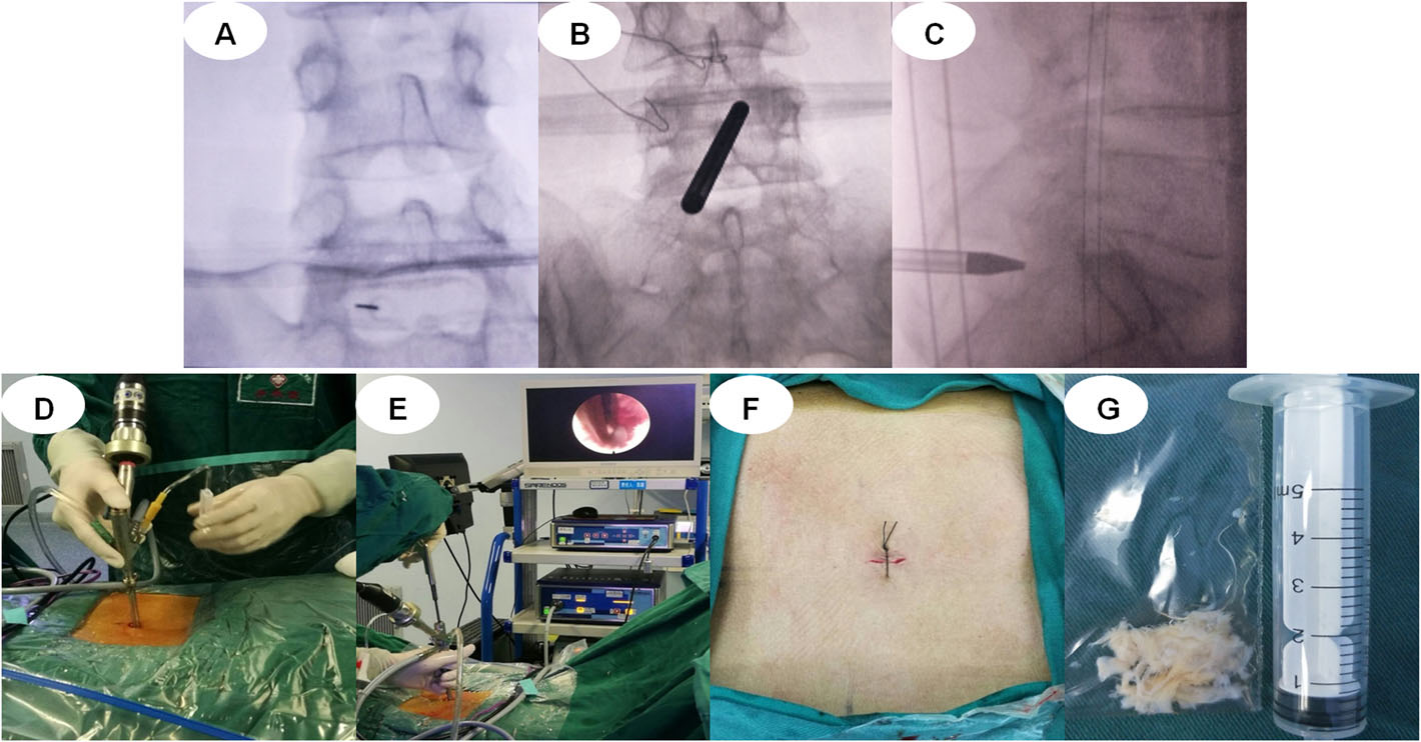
A typical PELD case via interlaminar approach. a puncture and radiography; b puncture and radiography; c puncture and radiography; d positioning of the tube; e the process of surgical operations; f the operative incisions; and g the herniated nucleus pulposus is removed. PELD, percutaneous endoscopic lumbar discectomy
